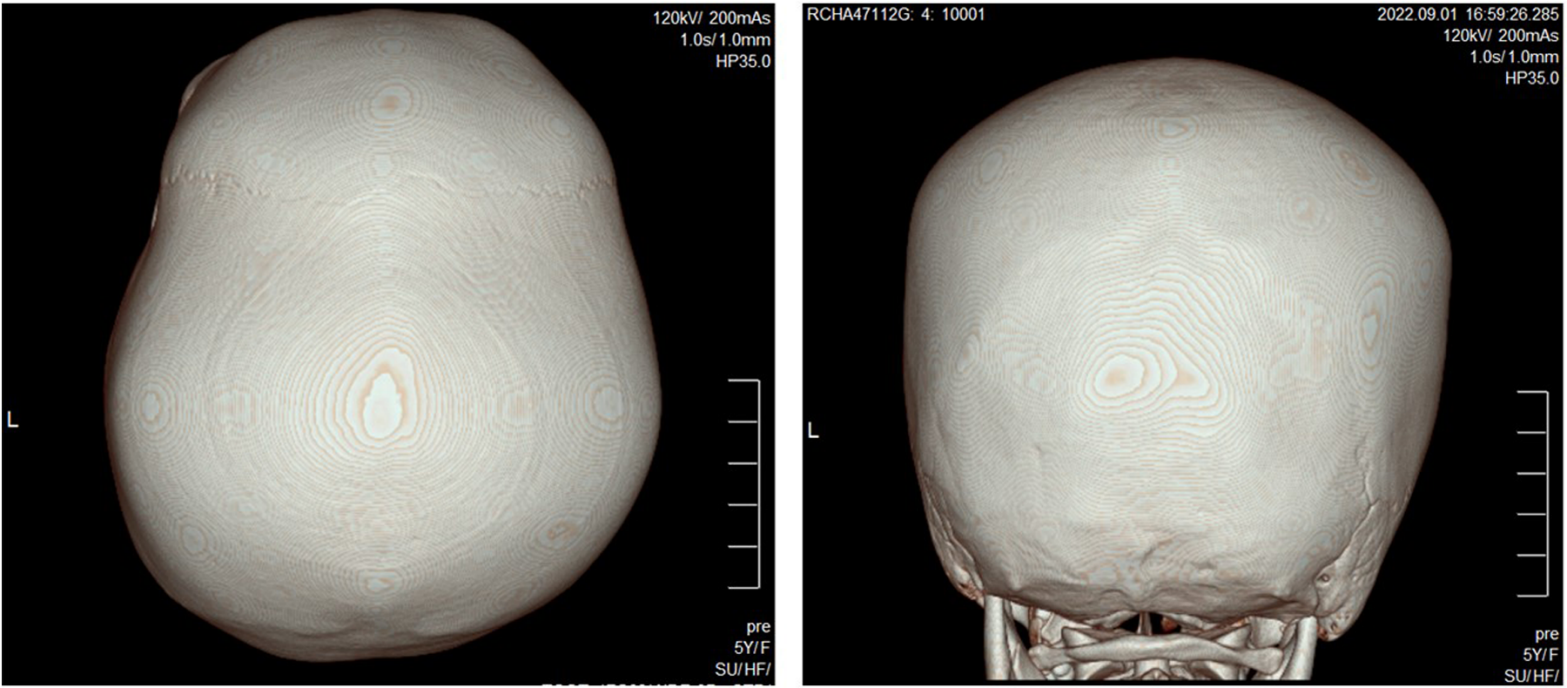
3D CT reconstruction of the skull shows closed sagittal and bilateral lambdoid sutures.
